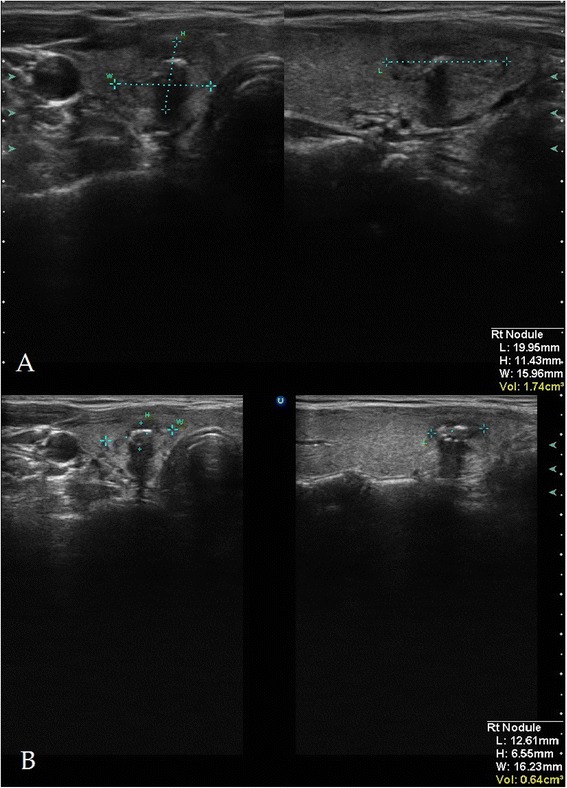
Volume measurement. Sonographical volume measurement of an exemplary thyroidal nodule before (A) and 3 months after (B) HIFU treatment.
